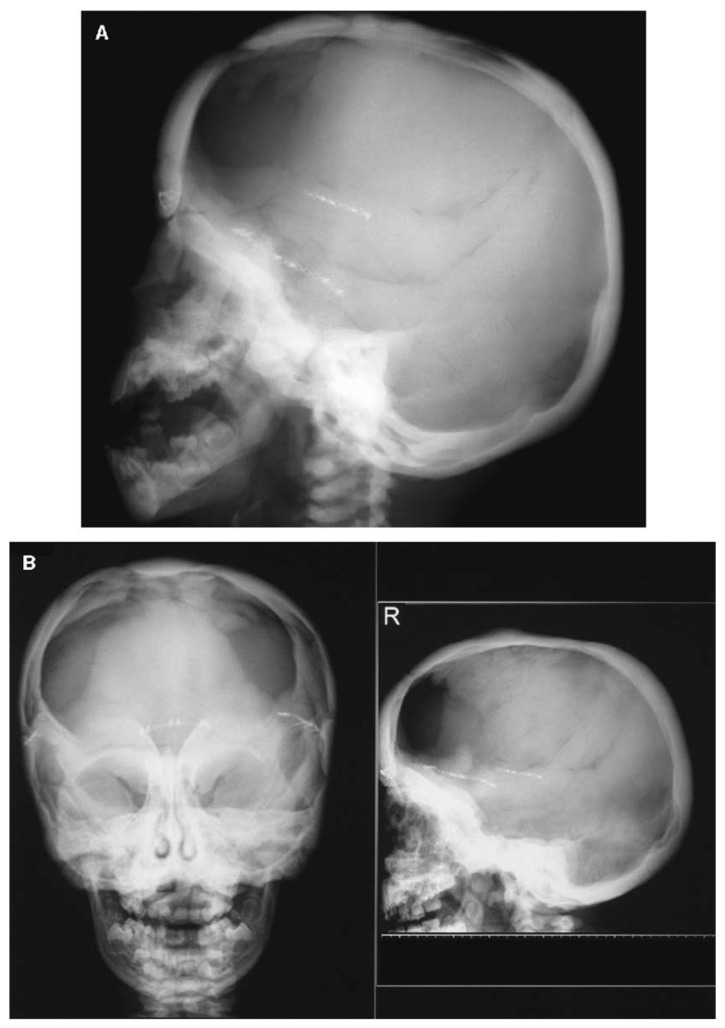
Cranial radiographs of a patient with a gain-of-function missense variant in *LRP5* taken at 1 year and 10 months of age (A) and at 3 years and 5 months of age (B). Progressive sclerosis of the cranial base and vault can be observed, and a craniotomy was performed at 11 months of age as a treatment for craniosynostosis. Adapted with permission from Ref. [54]. 2005, *Journal of Bone and Mineral Research*.
